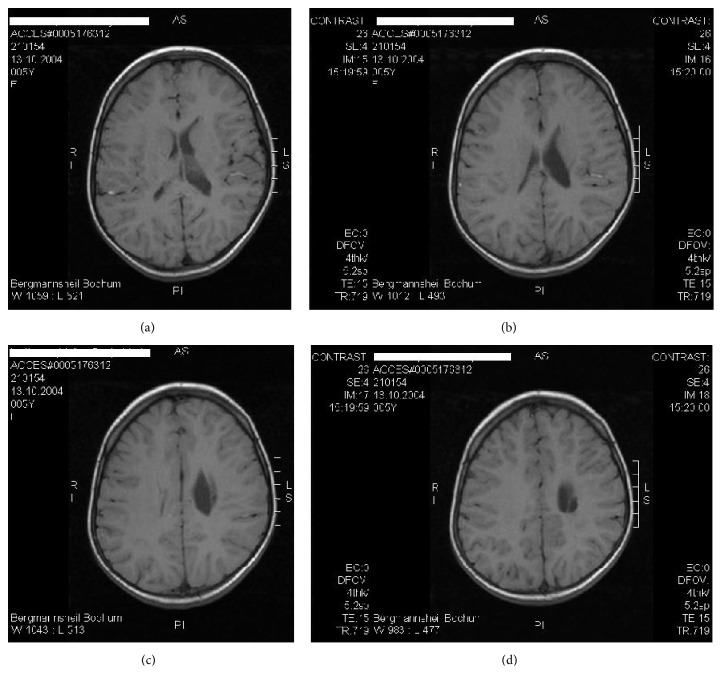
MRI of the brain (M.H.) at the age of 5 years (2009). Arterial ischemic stroke in the left central and paraventricular white matter with enlarged left ventricle before transplantation of autologous cord blood mononuclear cells (1.5-Tesla, head coil array, T1w TSE sequence).
